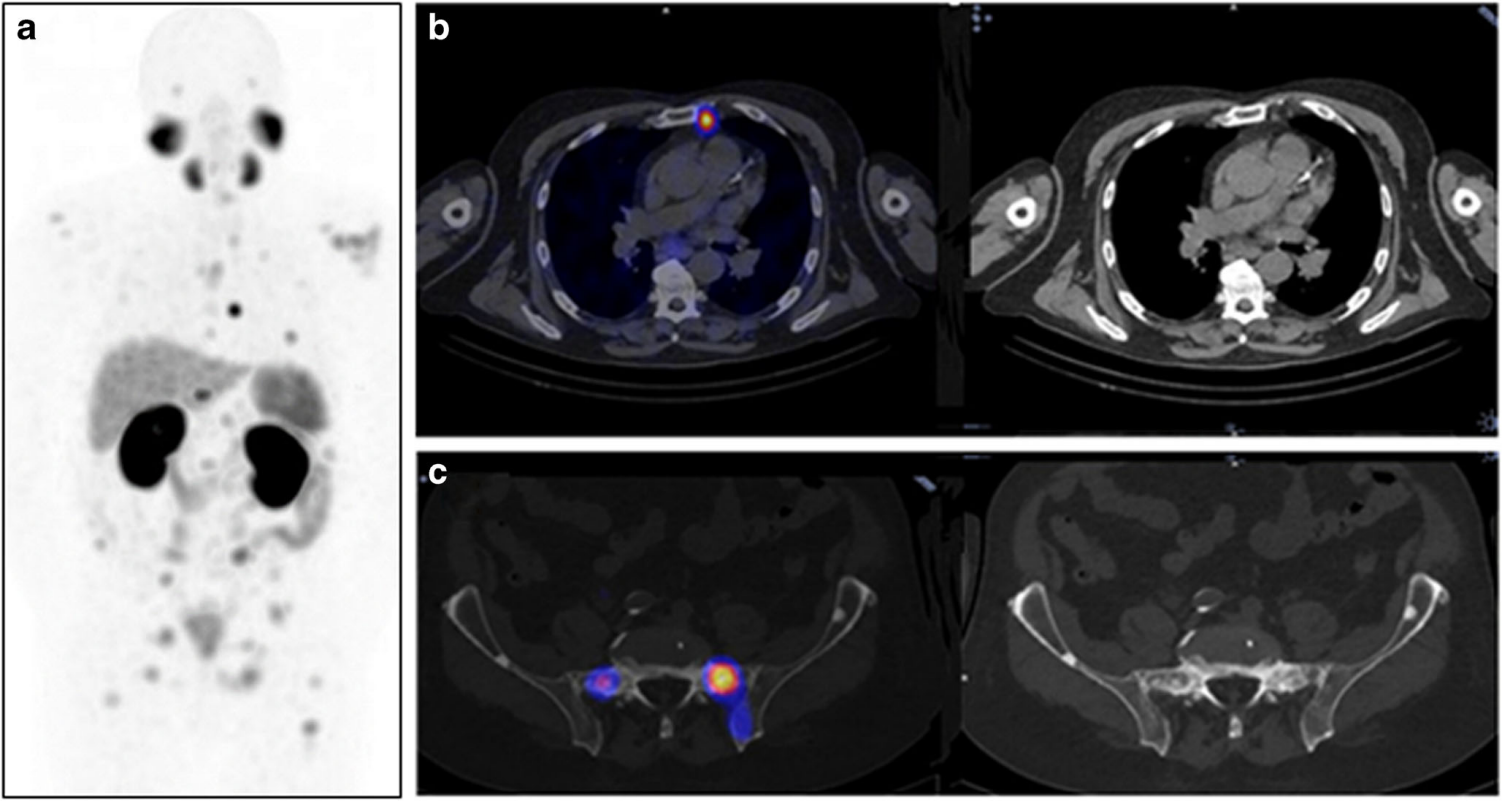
^99m^Tc-MIP-1404 PSMA-SPECT/CT in a patient with prostate cancer. An 81-year-old man with prostate cancer (pT4, N2, M0, G3) with biochemical recurrence diagnosed by increased serum PSA levels from 6.59 ng/mL to 17.34 ng/mL over a 3-month interval, was referred for restaging. Whole-body SPECT-MIP (a) shows multiple sites of focal abnormal tracer uptake above and below the diaphragm. Transaxial SPECT/CT and CT slices at the level of the upper thorax (b) demonstrate the presence of a left internal mammary chain lymph node metastasis. Transaxial SPECT/CT and CT slices at the level of the upper pelvis (c) show multiple, partially MIP-1404-avid osteoblastic lesions in the bone, consistent with functionally active and inactive skeletal metastases
